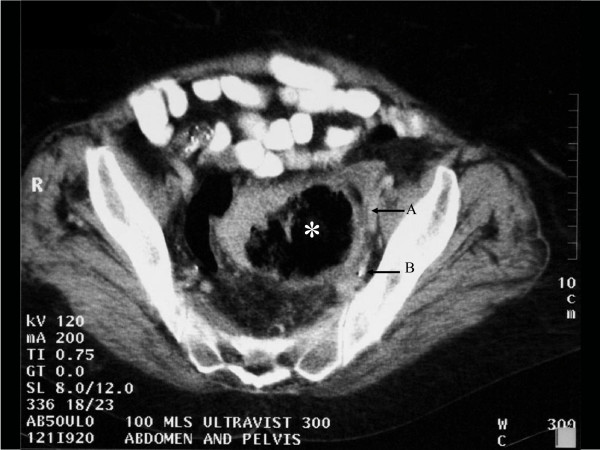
Axial computed tomography image with oral and intravenous contrast at the level of S1 demonstrating a giant colonic diverticulum*. The wall of the diverticulum and the surrounding fat are thickened denoting recent inflammation. Note the relationship to the left external and internal iliac vessels (arrows A and B, respectively). The obturator nerve (L2–4) emerges from the medial aspect of the psoas muscle and runs downwards and forwards, deep into [the internal iliac vessels.
